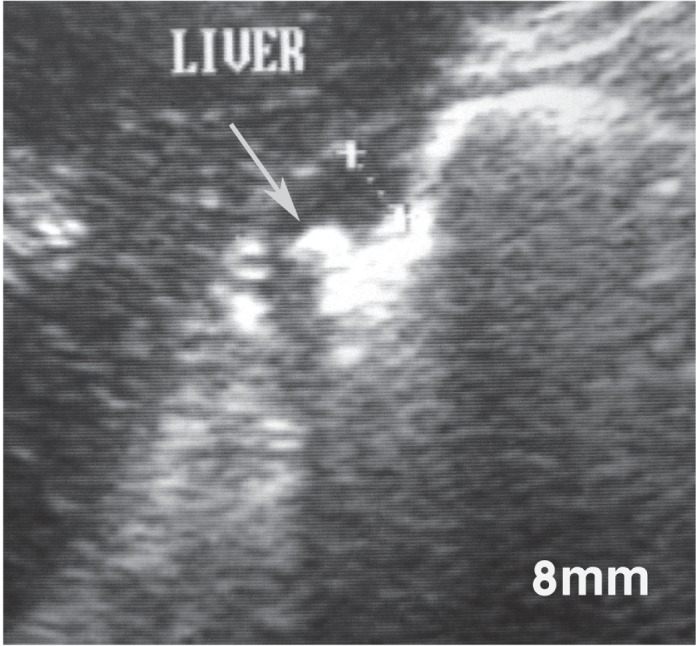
Abdominal ultrasound image in a 48-year-old female with benign lesser curvature gastric ulcer showing thickening of the gastric wall and a niche-like echogenicity (arrow), probably representing the ulcer carter.
